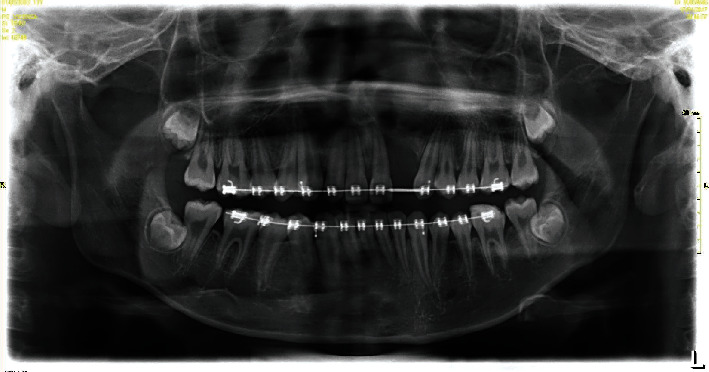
Panoramic radiograph after graft surgery.
